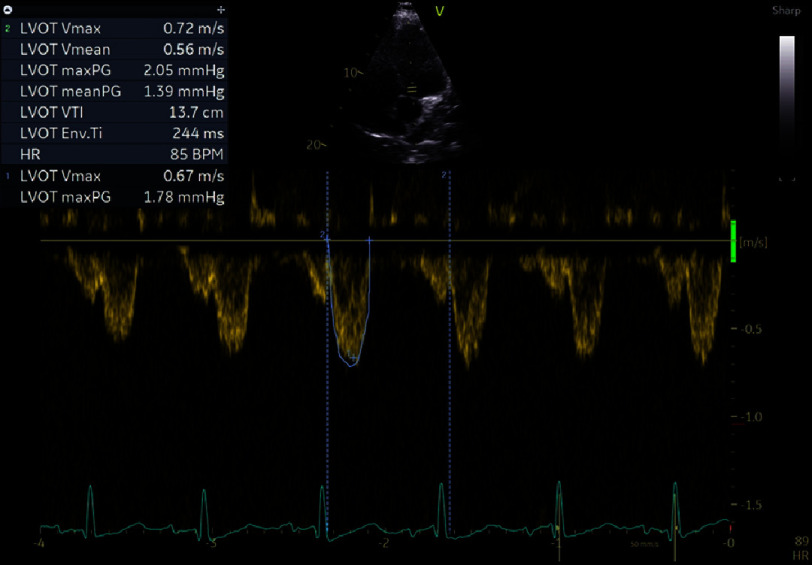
Initial echocardiography depicting reduced VTI in LVOTO, consistent with low cardiac output.
